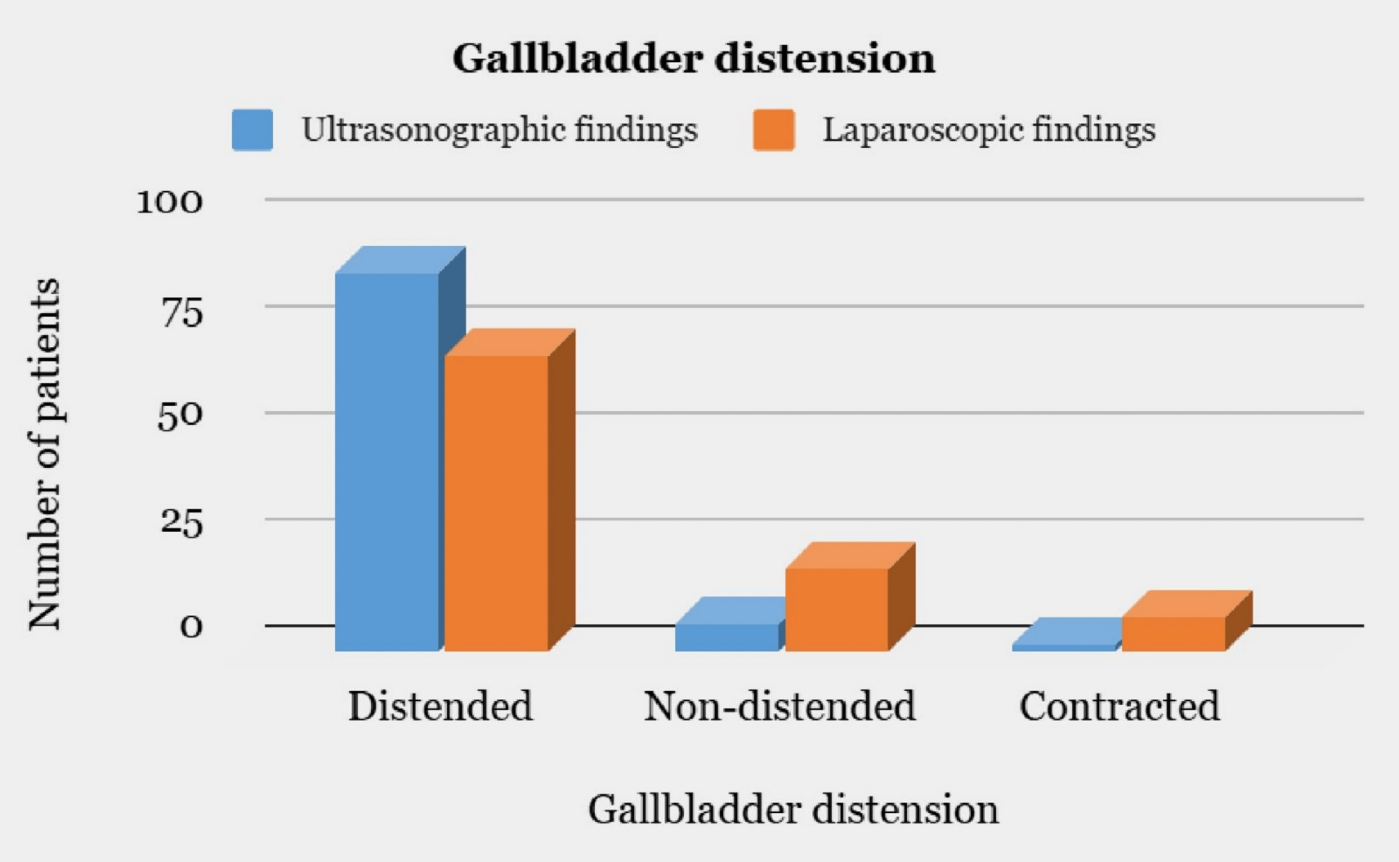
Comparison of ultrasonographic and laparoscopic findings with respect to gallbladder distension
